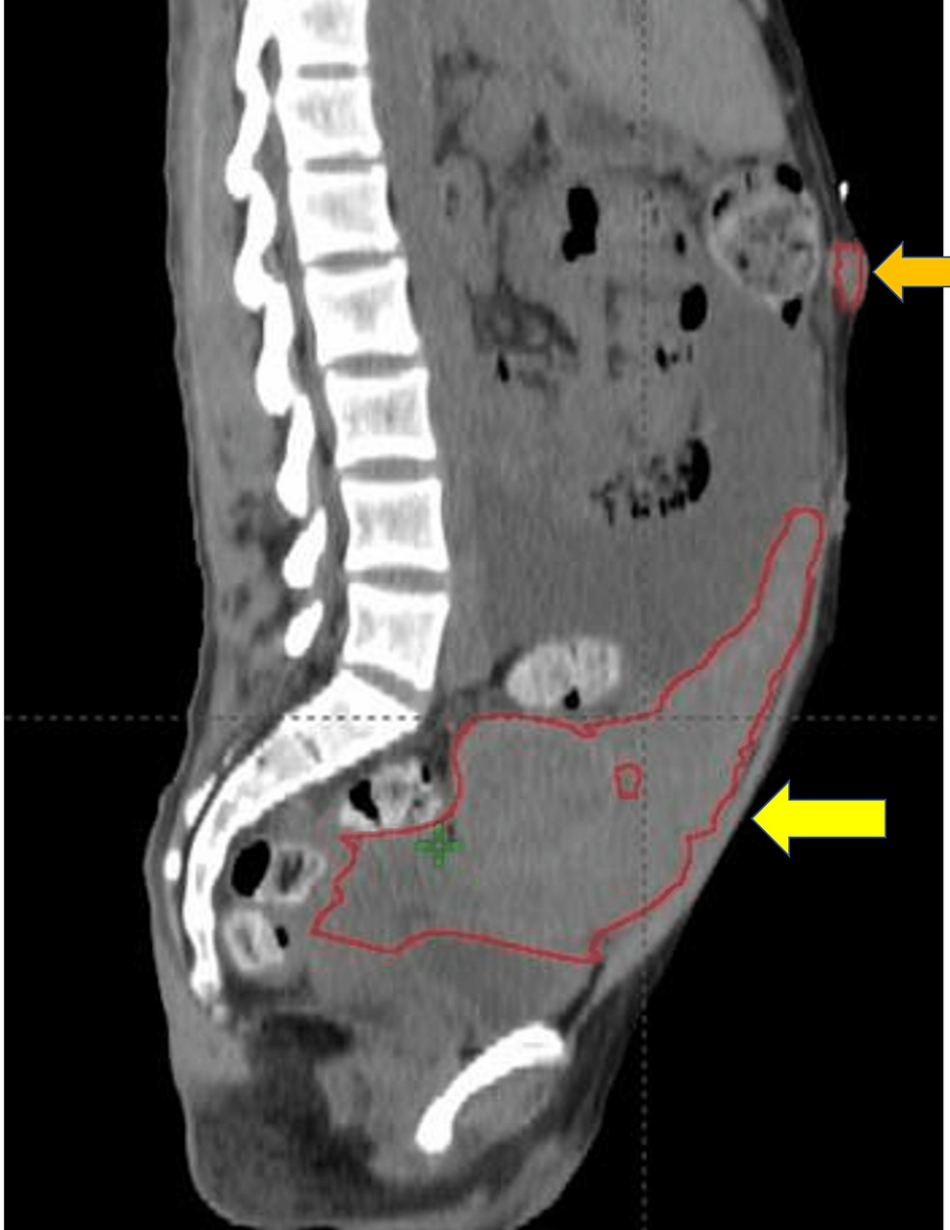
Sagittal CT with gross tumor volume (GTV) of subcutaneous nodule (orange arrow) and an abdominal and pelvic mass (yellow arrow).
